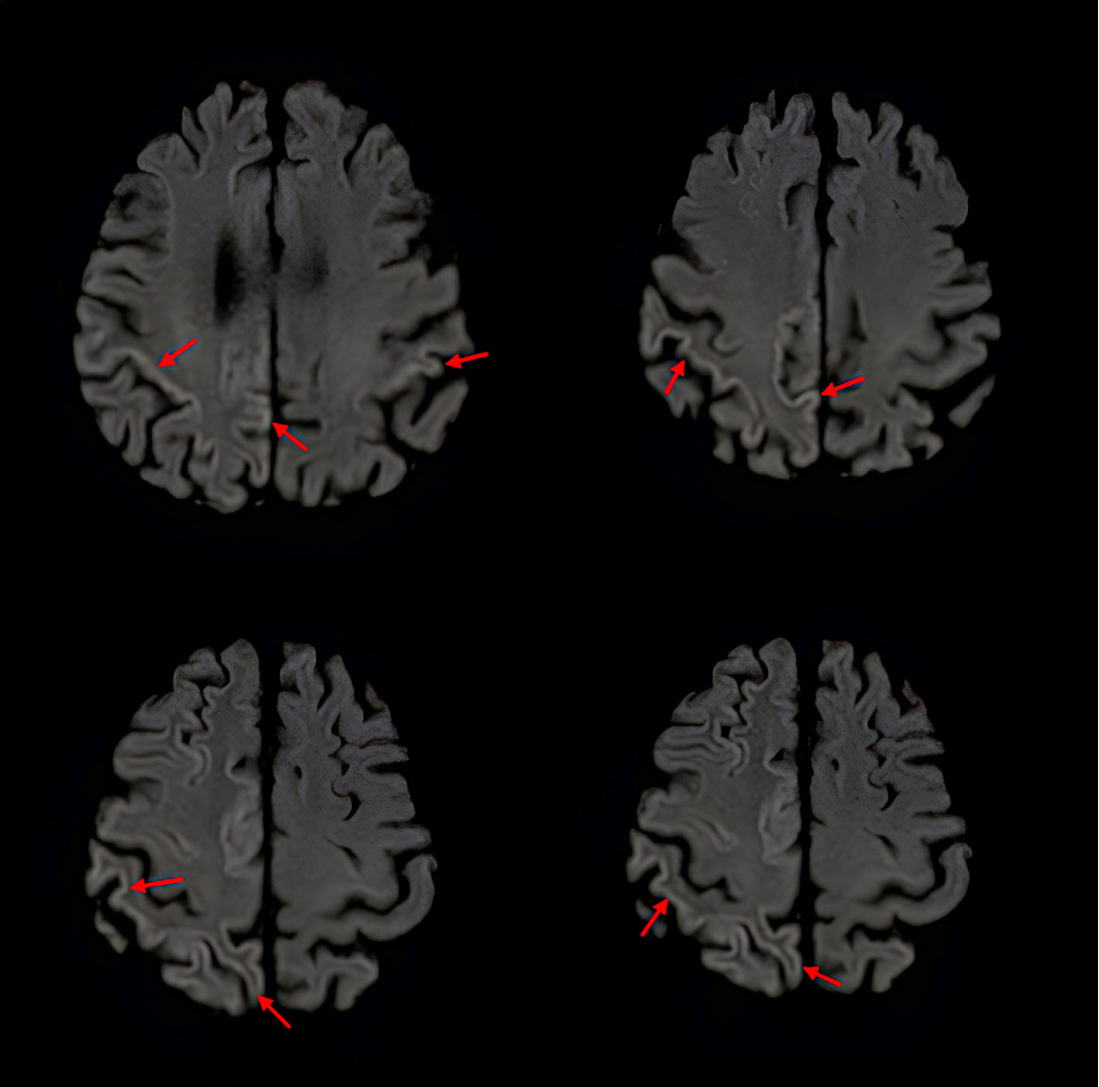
Diffusion‐weighted MRI (DWI) with right hemispheric and parietal accentuated diffusion restriction of the cortex (“cortical ribboning”). Specific anomalies are highlighted by red arrows.
